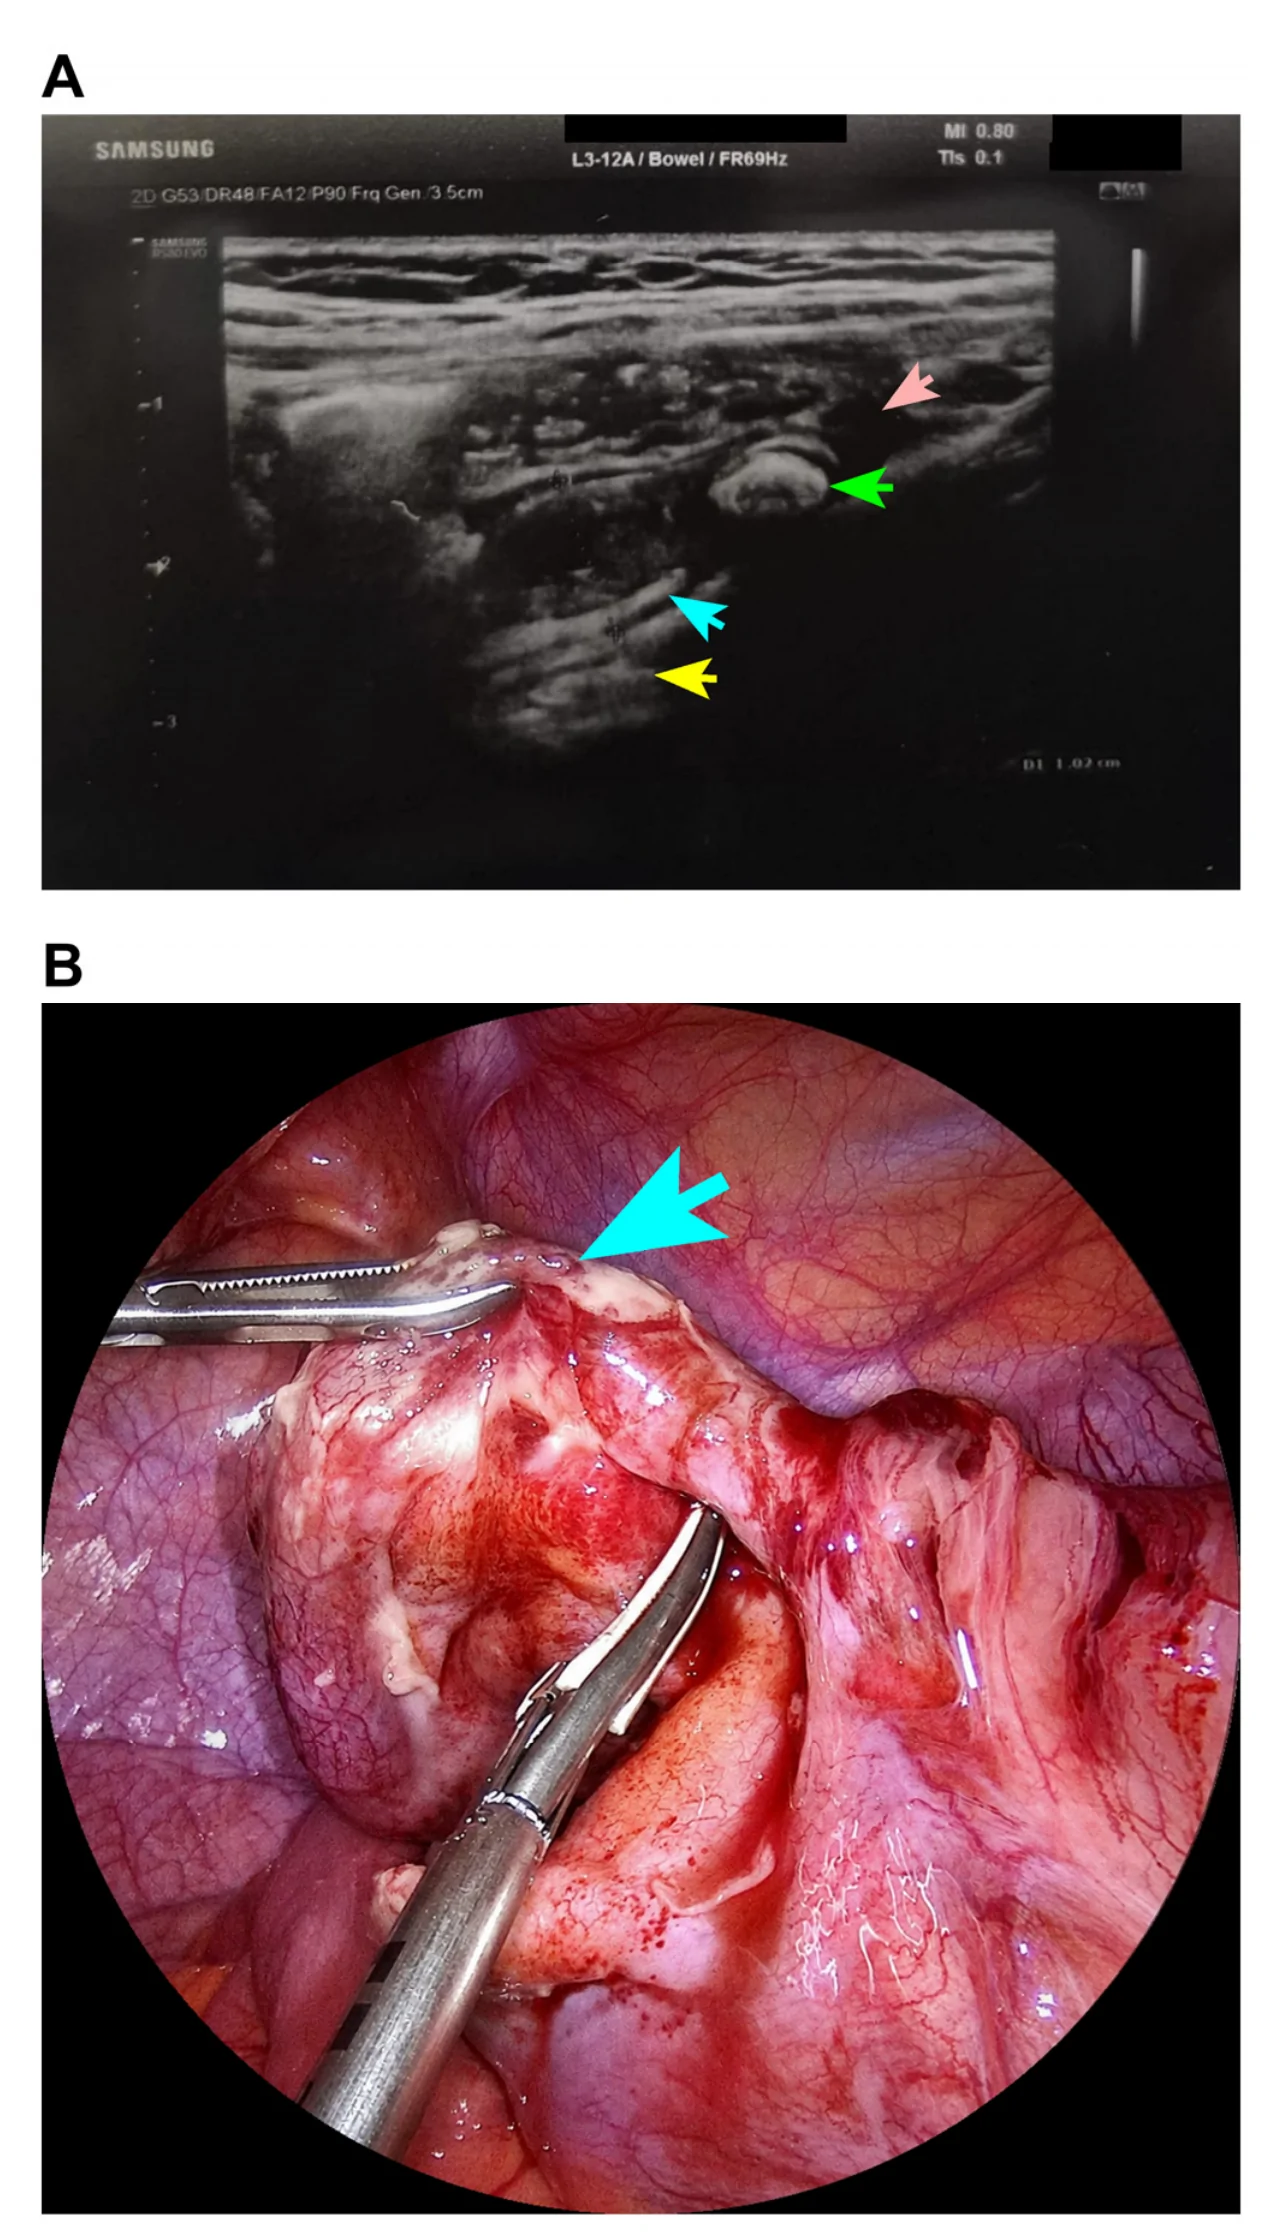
Representative preoperative sonographic and intraoperative laparoscopic findings in perforated appendicitis. (A) Sonographic image of the right lower quadrant, obtained with the linear L3-12A transducer (Section Ultrasound Assessment), showing an enlarged appendix with a maximum diameter of 1.02 cm (cyan arrow), a luminal appendicolith (green arrow), adjacent free peritoneal fluid (pink arrow), and increased echogenicity of the periappendiceal fat (yellow arrow). (B) Intraoperative laparoscopic view of the perforated appendix (cyan arrow), with gangrenous wall changes and associated peritonitis. The panels are from different patients. Patient identifiers have been removed.
